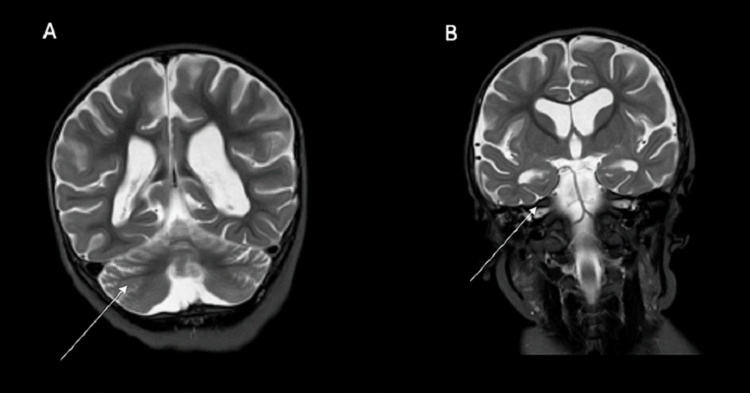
Panel A: T2 MRI sequence, coronal view; Panel B: T2 MRI sequence, coronal view Both images show cerebellar atrophy (white arrows).
